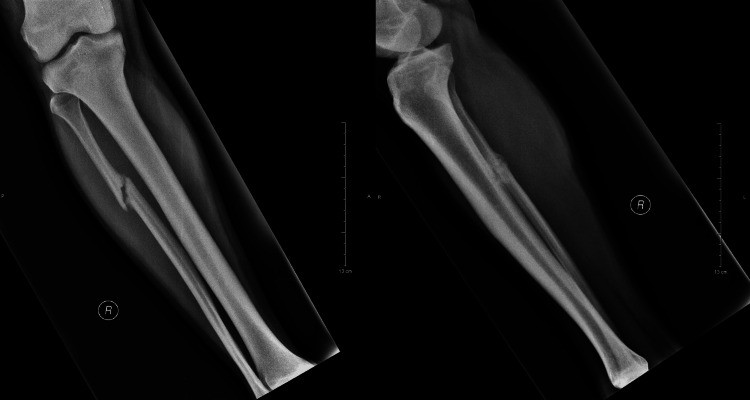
X-rays taken one month after the use of a weight-bearing cast
